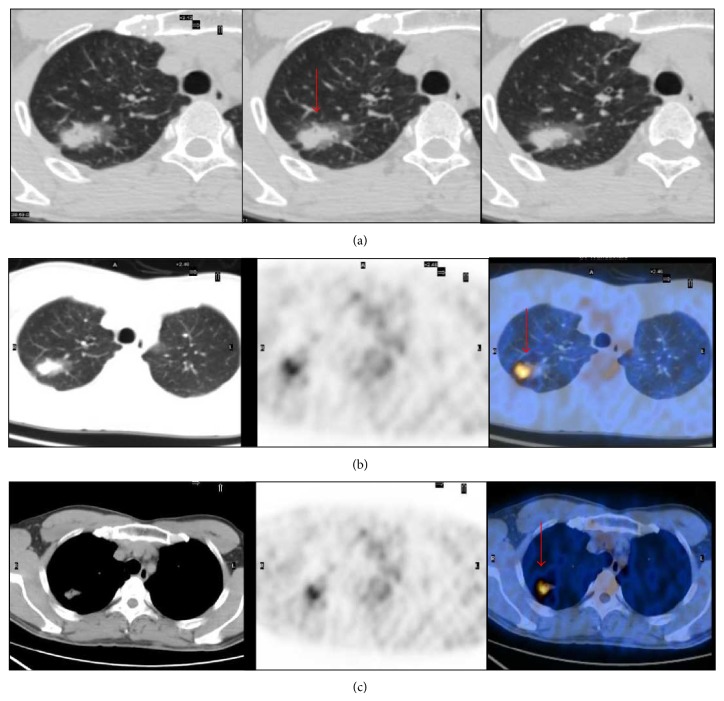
A 47-year-old male with an adenocarcinoma lesion with BAC features (red arrow). (a) Axial thin section CT image revealed a 2.3 cm mixed ground-glass opacity nodule in right upper lobe. (b, c) ^18^F-FDG PET/CT was positive for the lesion, and the maximum standardized uptake value = 3.59. Focal hypermetabolism was localized in the soft part of the lesion but not in the ground-glass part.
